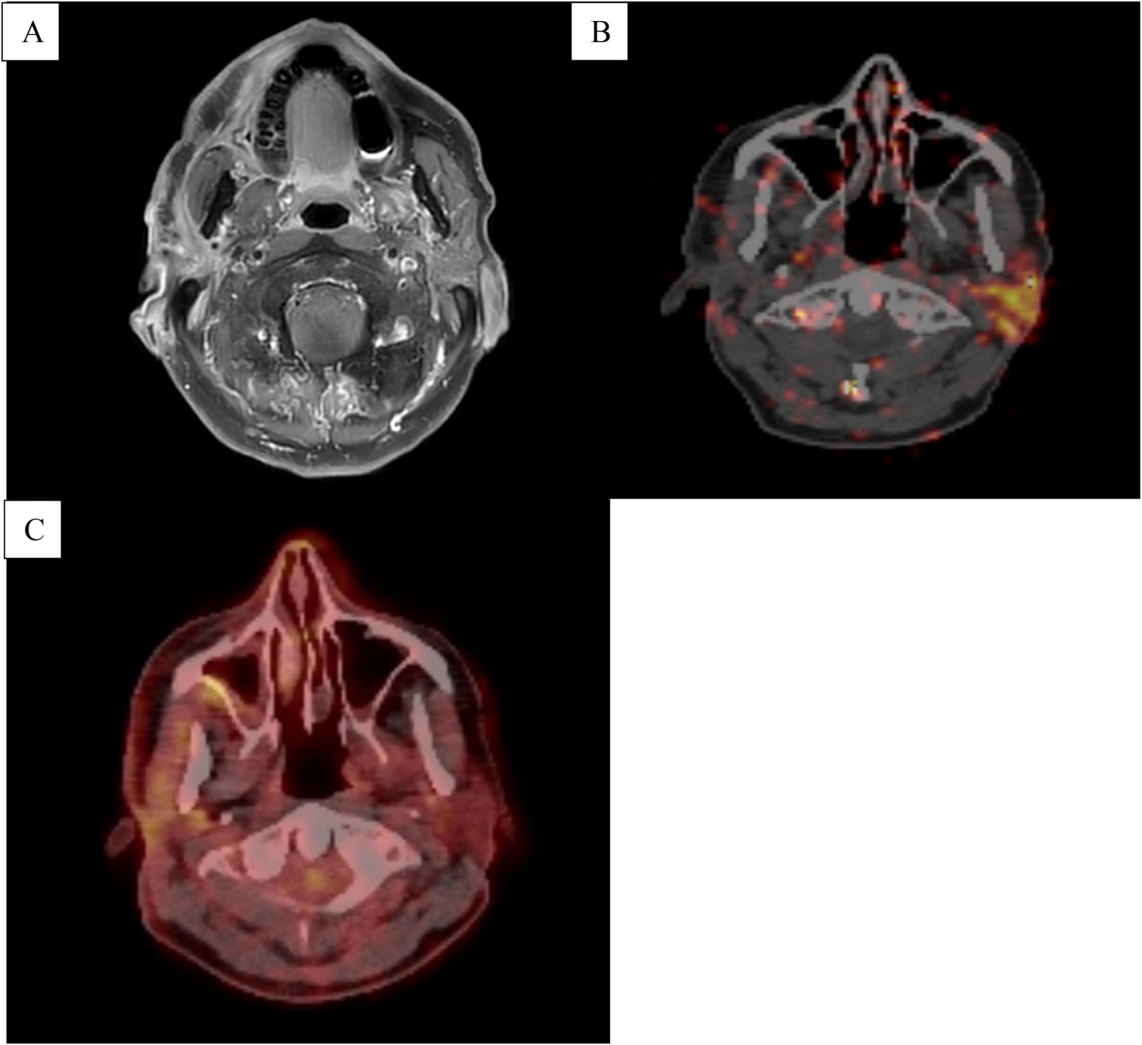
(A) T1-weighted MRI scan of the neck with contrast revealing a hyperintense diffuse lesion of the right parotid gland. (B) PSMA-PET/CT scan demonstrating tracer uptake in the left parotid gland and a lack of tracer uptake in the right parotid gland suggestive of tumor-destructed glandular tissue. (C) FDG-PET/CT scan depicting low-level, focally increased tracer uptake of the right parotid gland.
